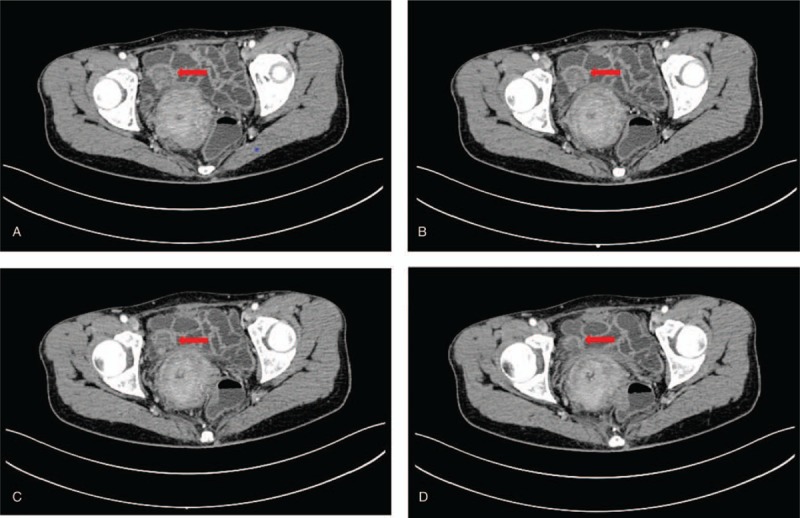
Abdominal enhanced computed tomography showed a soft tissue mass shadow with mild delayed enhancement extended into the distal ileal lumen (A, B, C, D). The narrow indicates the tumor.
